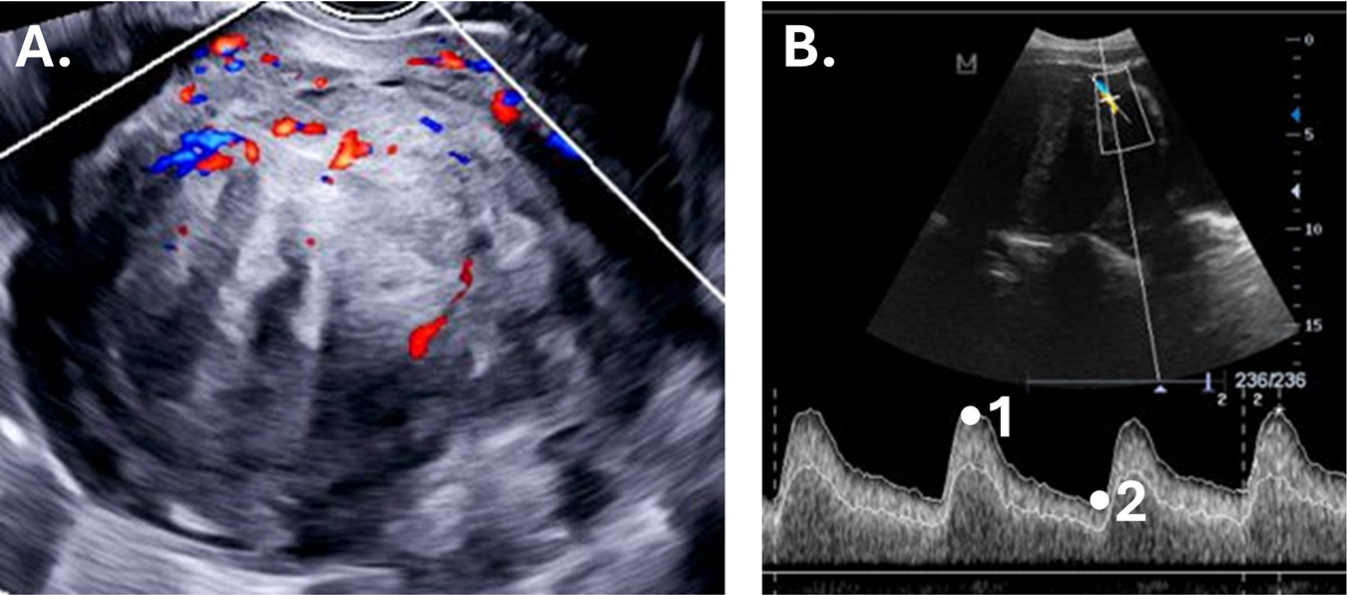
Sagittal fibroid images from patient 2 obtained by 2D Color Doppler ultrasound (A), including color hues reflecting location and direction of blood flow; and 2D Spectral Doppler ultrasound (B) from patient 3 [80], including the gate to detect Doppler shift and showing blood flow velocity (*y*-axis) plotted against time (*x*-axis), resulting in a peak systolic velocity (1) and end diastolic velocity (2)
